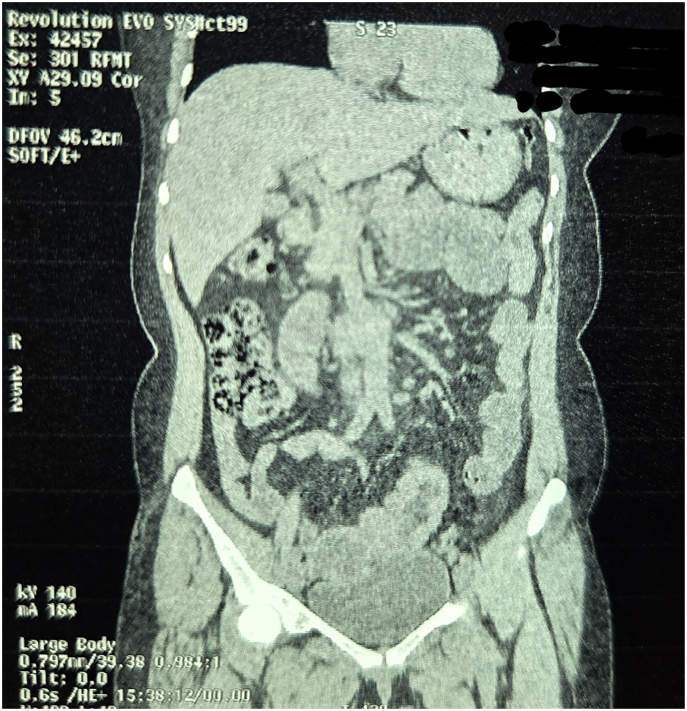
Non Contrast Computed Tomography of Abdomen and Pelvis (Coronal Section Zoomed In).
